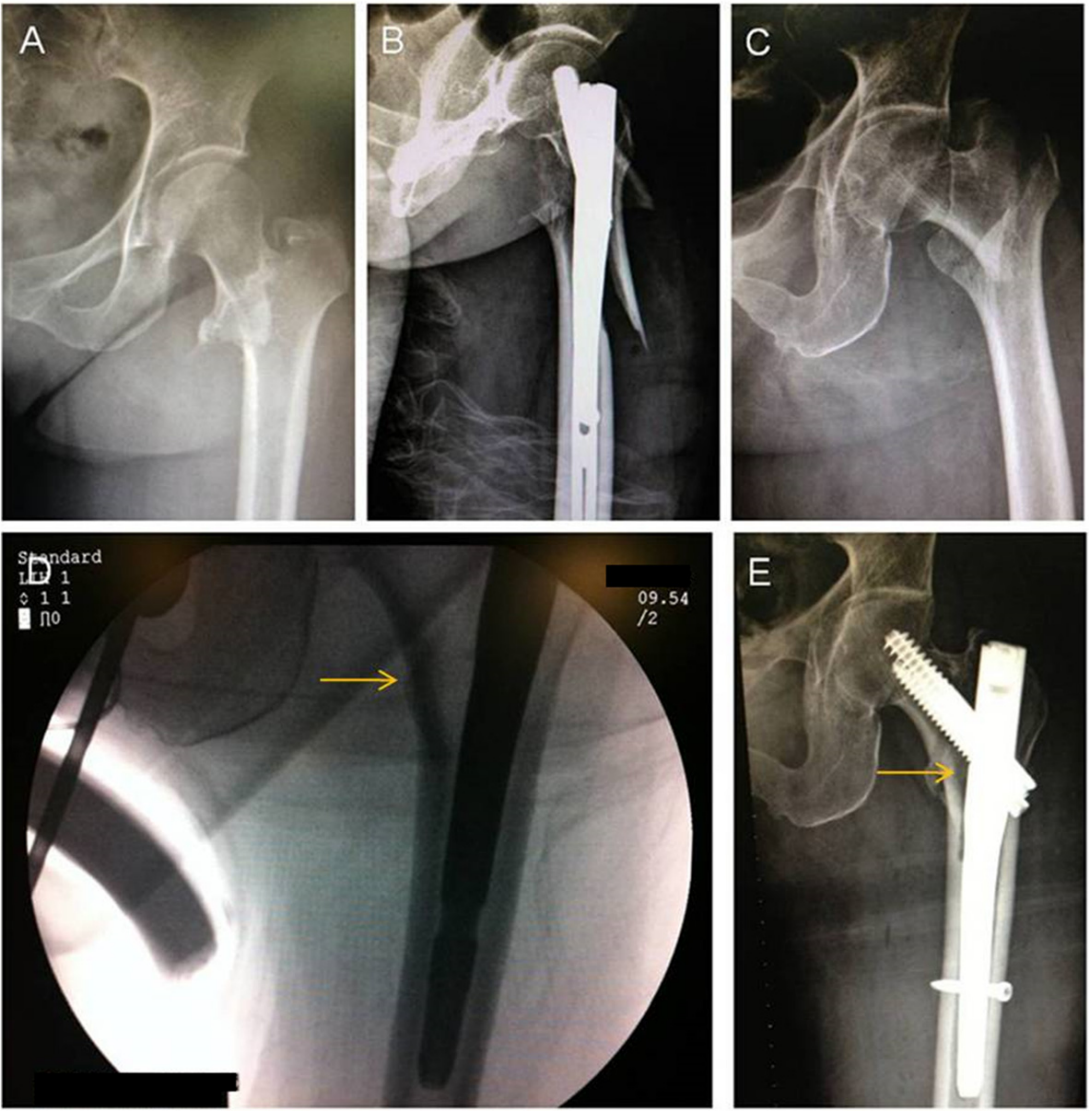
Preoperative & postoperative anterior and posterior x-ray images of the injured hip with INTERTAN™ nails resulting in iatrogenic fractures in an obese patient then fixed with short and long INTERTAN™ nail. (A) Preoperative anteroposterior X-ray image of the injured hip. (B) Postoperative anteroposterior X-ray image of the injured hip, showing the iatrogenic fractures of the proximal femur (C). Preoperative anteroposterior X-ray image of the injured hip of another obese patient (D). Based on our experience we kept the vertical trajectory with the nail insertion and fully reamed the medullar canal of the proximal femur, of the case in Figure 2A/B, with the anatomical specificity of an extremely narrow proximal canal (arrow). (E) With this improvement, a relatively smooth insertion was achieved (arrow).
